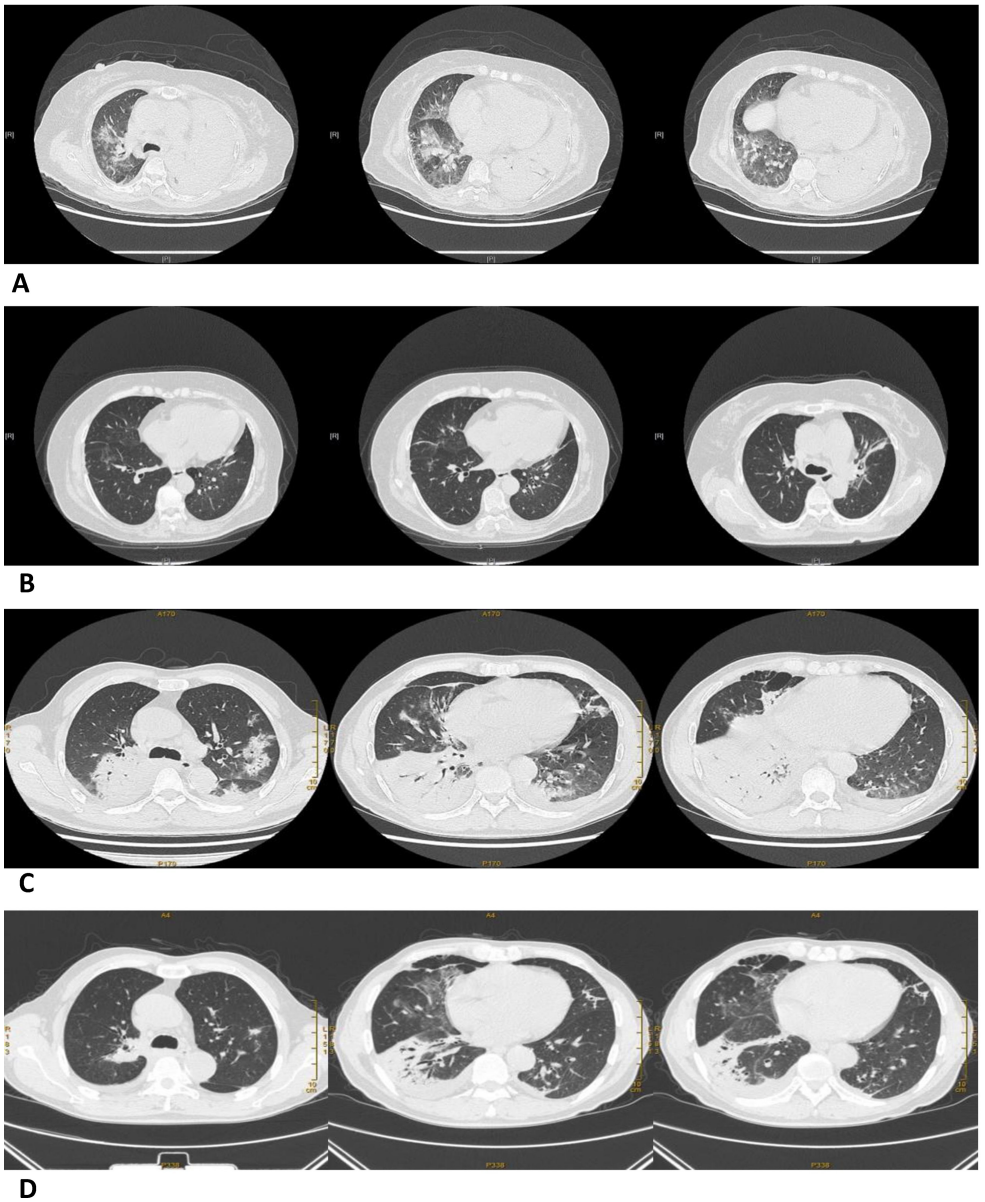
Pulmonary CT images before and after treatment in a severe and a common *Chlamydia psittaci* pneumonia patient. (A) Large consolidation of the left lung and patchy exudation shadow of the right lung before treatment (07/02/2021). (B) The area of consolidation and patchy exudation shadow significantly reduced after treatment (01/03/2021). Pulmonary CT images before and after treatment in a common *Chlamydia psittaci* pneumonia patient. (C) Large areas of exudation and consolidation in the right upper lobe and right lower lobe, bronchial inflation signs, and minor pleural effusion before treatment (18/09/2020). (D) Lesion clearly absorbed after treatment (29/09/2020).
